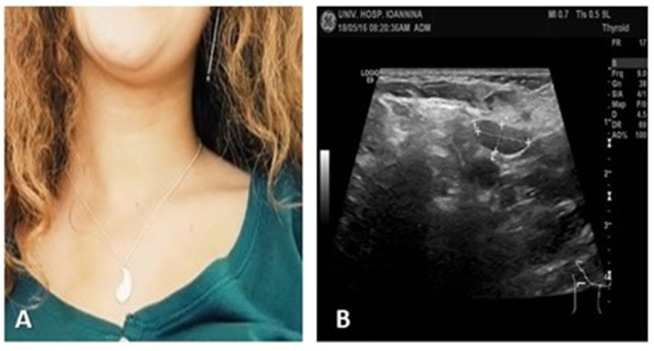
(A) Patient in follow-up, without lymphadenopathy; (B) ultrasound: 2 small lymph nodes with normal architecture and echo structure.
